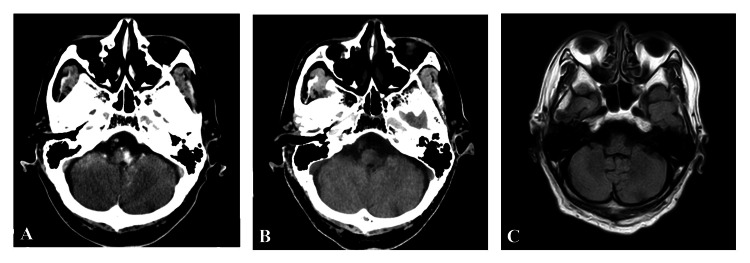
Post-operative images of Case 2 A: Plain CT immediately after the procedure shows a high-density lesion in the left lateral medulla oblongata. B: Water-iodine image of dual-energy CT shows no lesions. C: T2 image of MRI obtained one day after the procedure shows no lesions.
